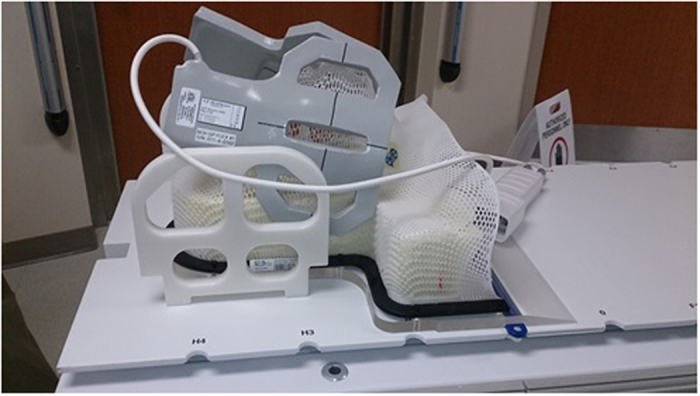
Patient immobilization device and magnetic resonance imaging (MRI) coil arrangement. Imaging patients while immobilized in the position in which they would receive radiation treatment drastically reduces motion artifact, enabling the acquisition of high-quality chemical exchange saturation transfer (CEST) and other MR images.
